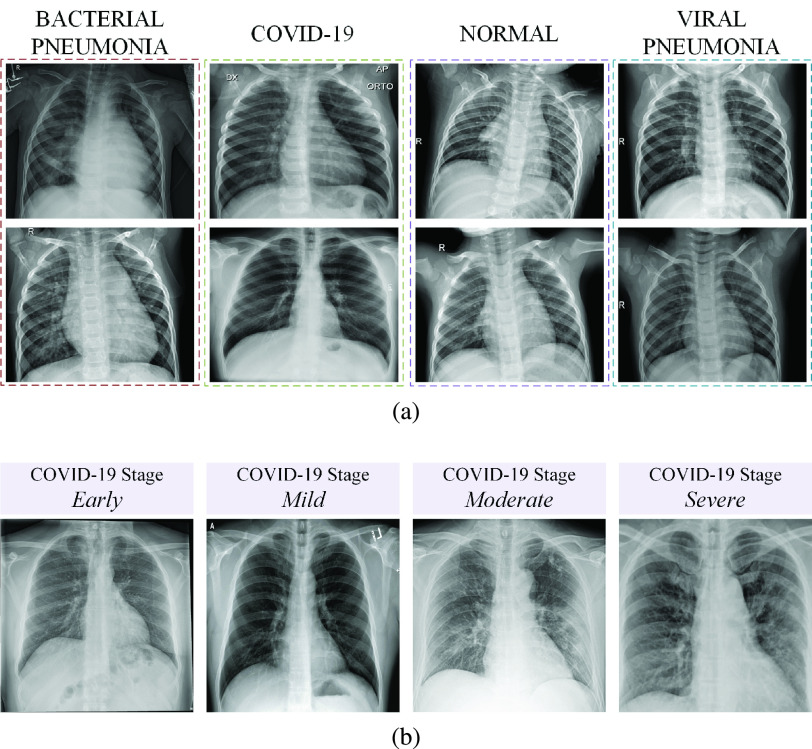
Sample QaTa-Cov19 X-ray images. (a) X-ray images from different classes. (b) X-ray images from the COVID-19 patients who are in the different stages.
